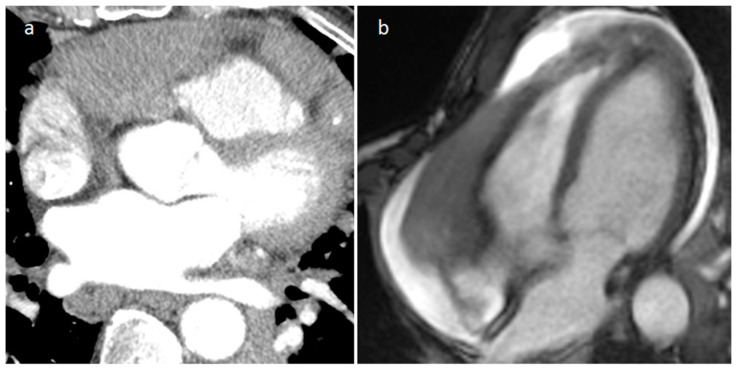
Right atrioventricular groove IgG4-related disease on arterial CT phase (a) and on cine MR sequence (b).
